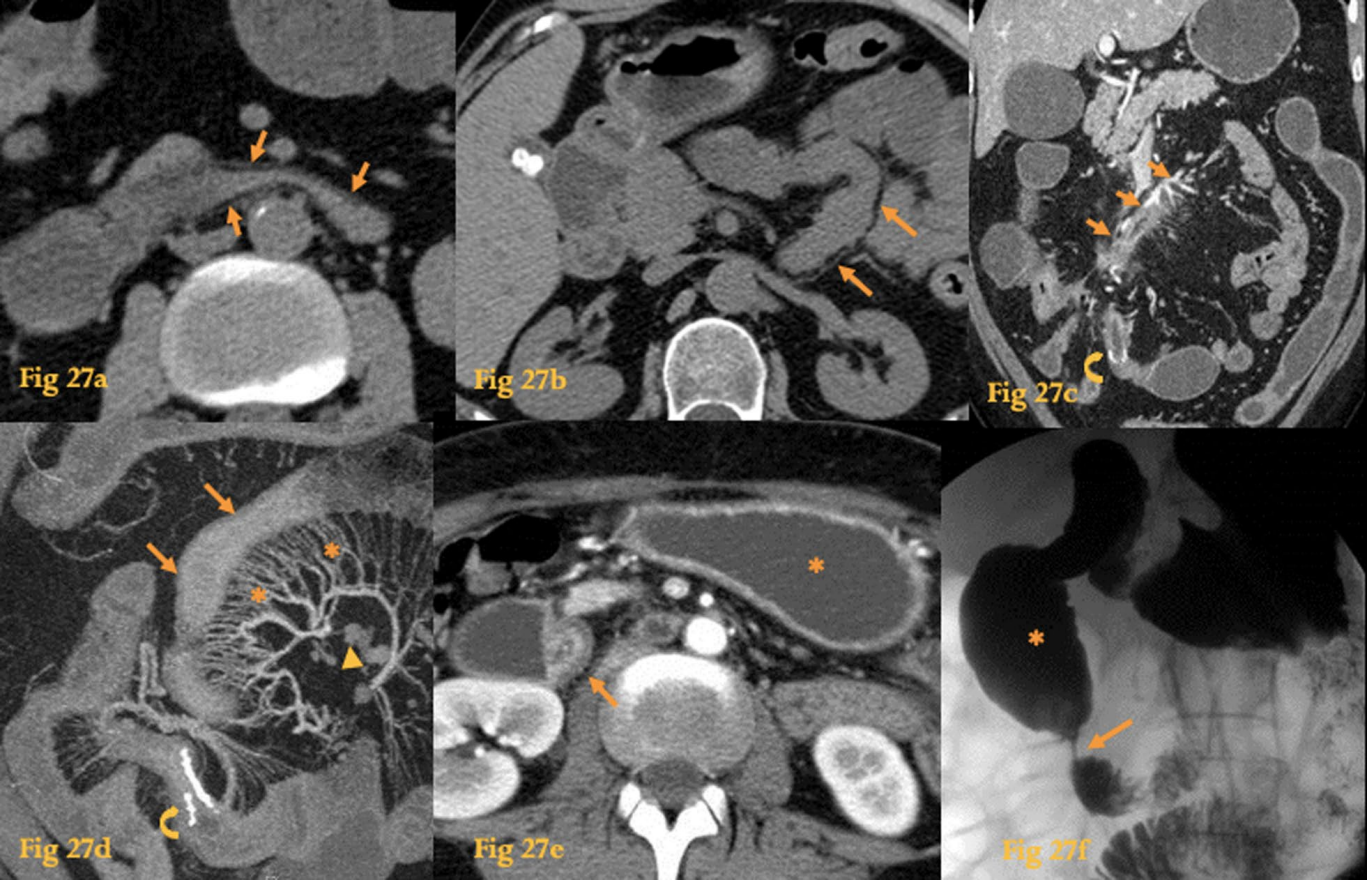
Chron’s Disease. Plain abdominal CT shows the fat halo sign (arrows) in the wall of the D3 (a) and D4 (b), in a patient with quiescent Crohn’s disease. CT enterography (c) of a CD patient with active disease who previously had a segmental enterectomy—note the surgical clips at the ileal-ileal anastomosis (curved arrows). There is a fistula between the thickened ileal segment near the anastomosis and the duodenum. MIP coronal reformation (d) shows mesenteric engorgement (asterisks) and adenopathy (arrowhead) near a thickened bowel wall segment (arrows). Abdominal CT of another patient with active disease (e) shows gastric outlet obstruction (asterisk) and a stricture in D2 that shows the target sign (arrow). Barium study of the same patient (f) shows the short stricture (arrow) and dilatation of the proximal duodenum (asterisk)
